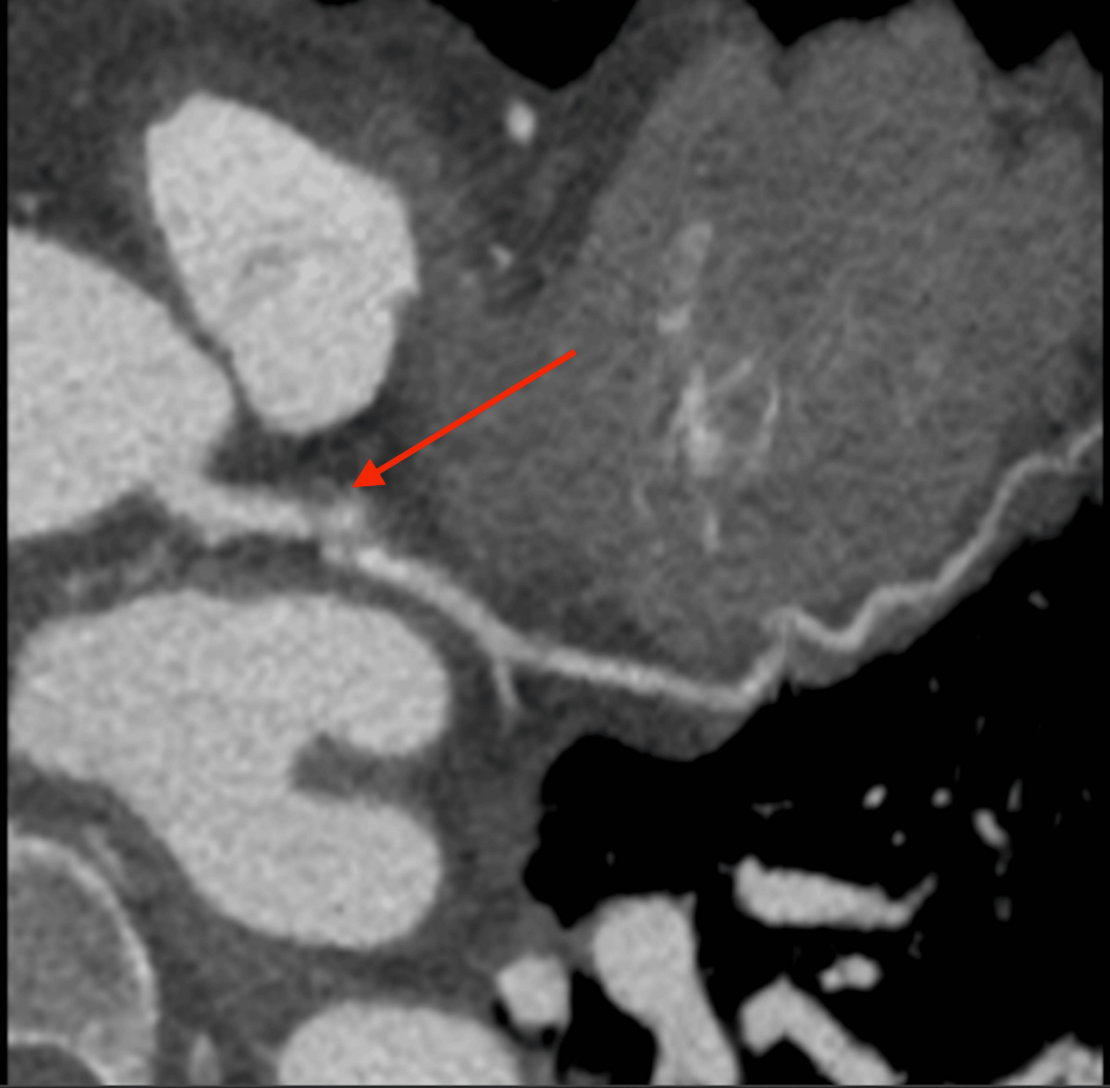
Computed tomographic angiogram of coronary arteries showed an 11 mm long by 9 mm wide nonthrombosed aneurysm emanating out of the distal end of the left main coronary artery to the proximal left circumflex. The red arrow depicts the left main coronary artery bifurcating to the proximal left circumflex.
